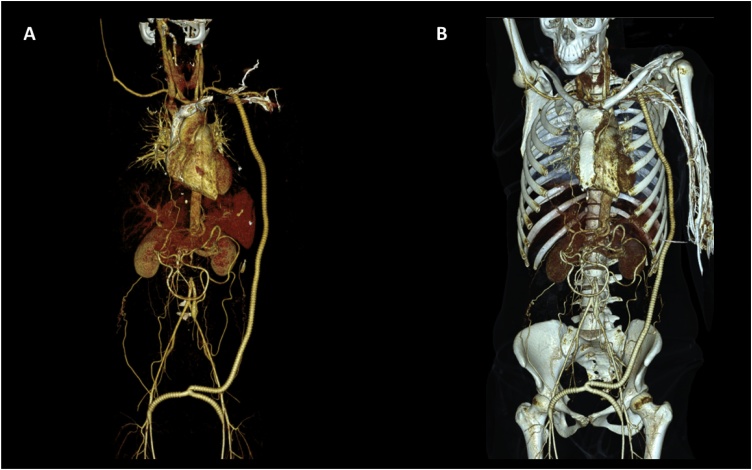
Post-surgical abdominal angiotomography showing a permeable axillobifemoral prosthetic graft. A and B) 3-D reconstruction computed tomography.
